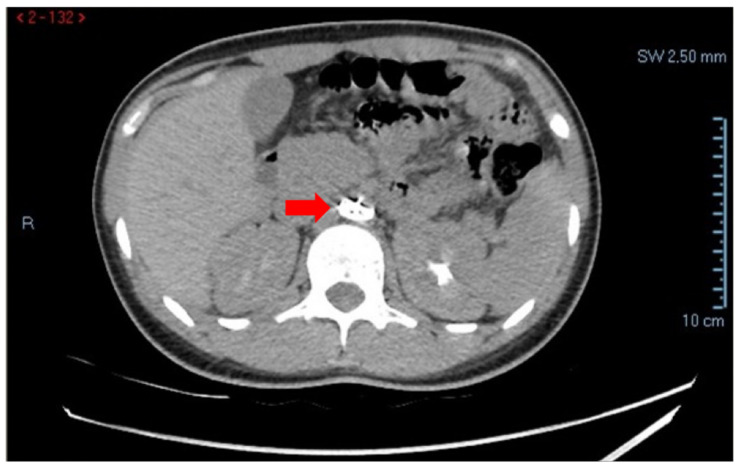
Contrast-enhanced CT of the abdomen demonstrating migration of the Amplatzer occluder into the infra-renal abdominal aorta (arrow), incidentally detected during evaluation for acute abdominal pain.
